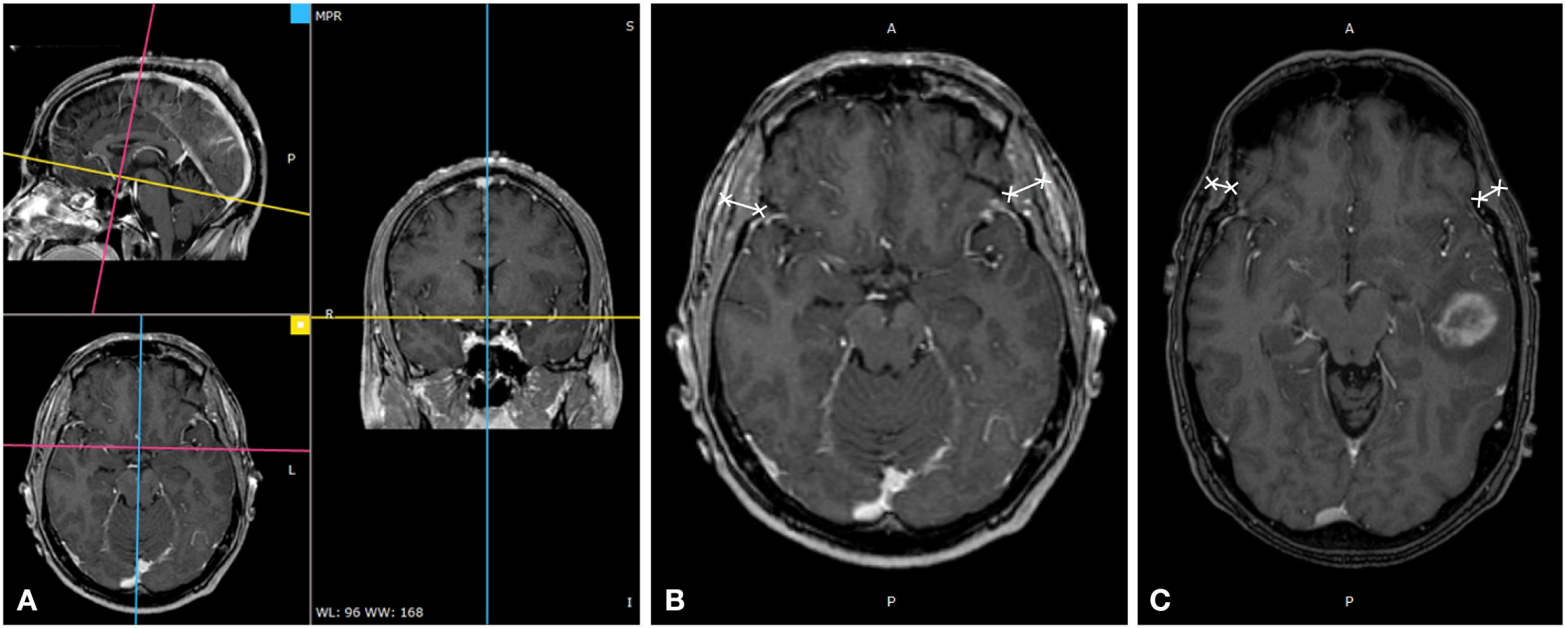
Temporal muscle thickness (TMT) measurement on cranial MRI. (A) TMT measurement on cranial thin T1WI contrast-enhanced image through multiplanar reformation, (B) A 49-year-old male patient with an overall survival of 38.1 months (bilateral mean TMT = 10.20 mm), and (C) a 57-year-old male patient with an overall survival of 16.9 months (bilateral mean TMT = 5.94 mm).
